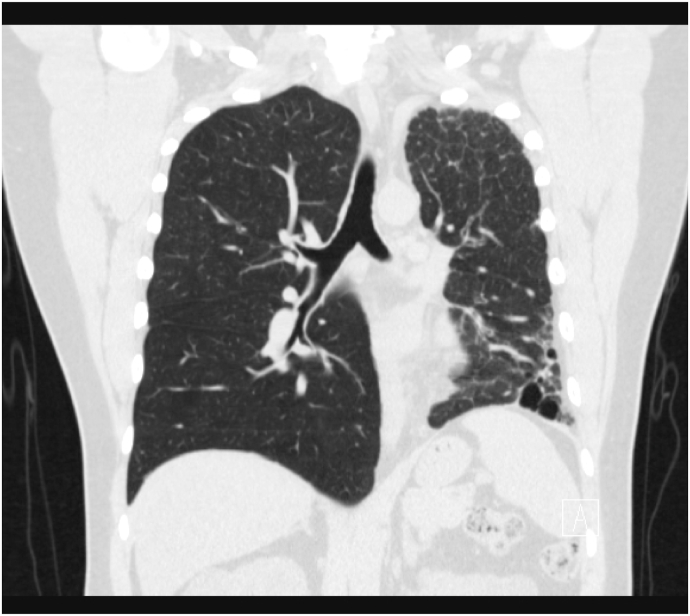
CT scan of the chest showing a hypoplastic left lung with basilar reticulations, pulmonary fibrosis, and cystic airspace disease with a hypoplastic left pulmonary artery.
